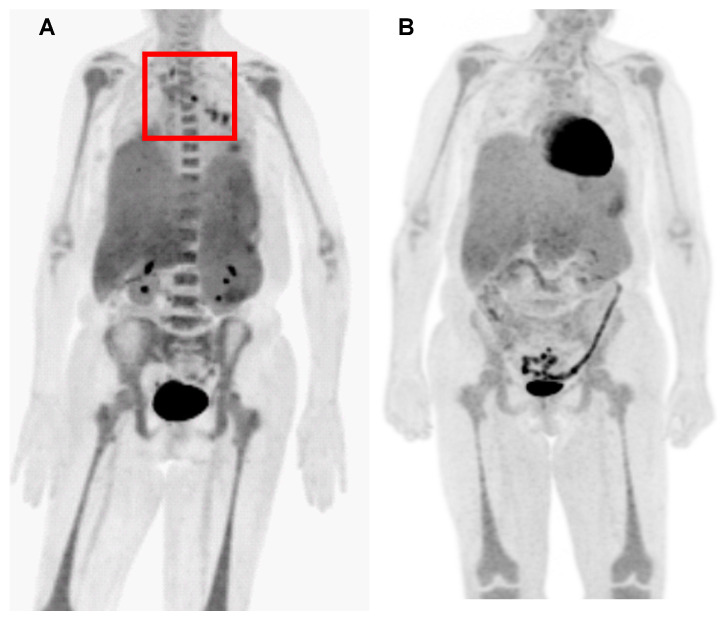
Serial PET scan in our patient with disseminated histoplasmosis. (A) PET showed newly developed mediastinum lymphadenopathy (red square) while the patient was receiving intravenous liposomal amphotericin B (3 mg/kg/daily) and oral itraconazole. (B) After switching to posaconazole and increased liposomal amphotericin B (5 mg/kg/daily), follow-up PET showed decreasing F-18 FDG avidity of the mediastinal and hilar lymph nodes. Abbreviation: PET—positron emission tomography.
